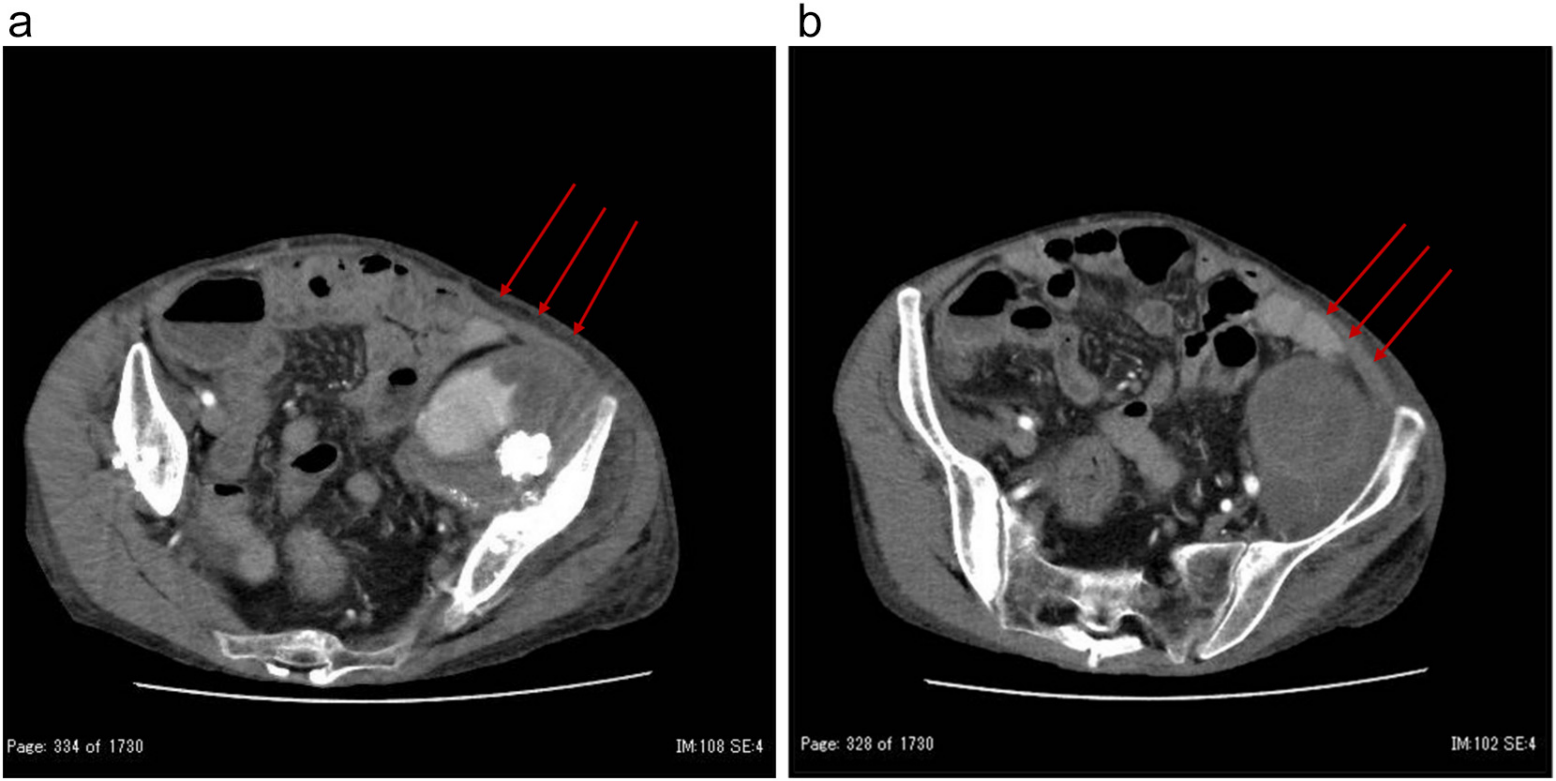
(A, B) Computed tomography revealed suspected rupture of the external iliac artery.
